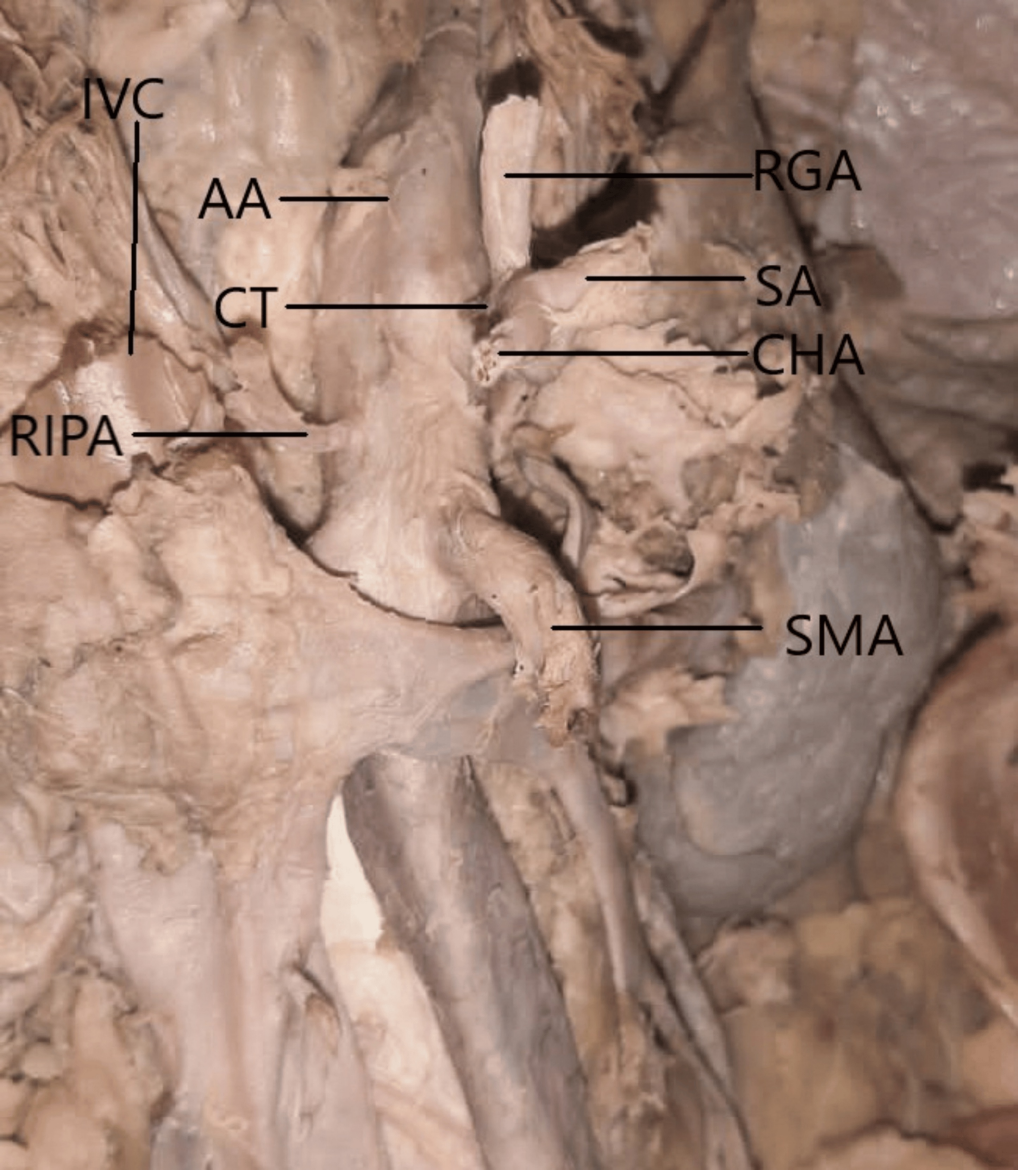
Origin of the RIPA The RIPA had a normal origin. AA, abdominal aorta; CHA, common hepatic artery; CT, coeliac trunk; RGA, right gastric artery; RIPA, right inferior phrenic artery; SA, splenic artery
